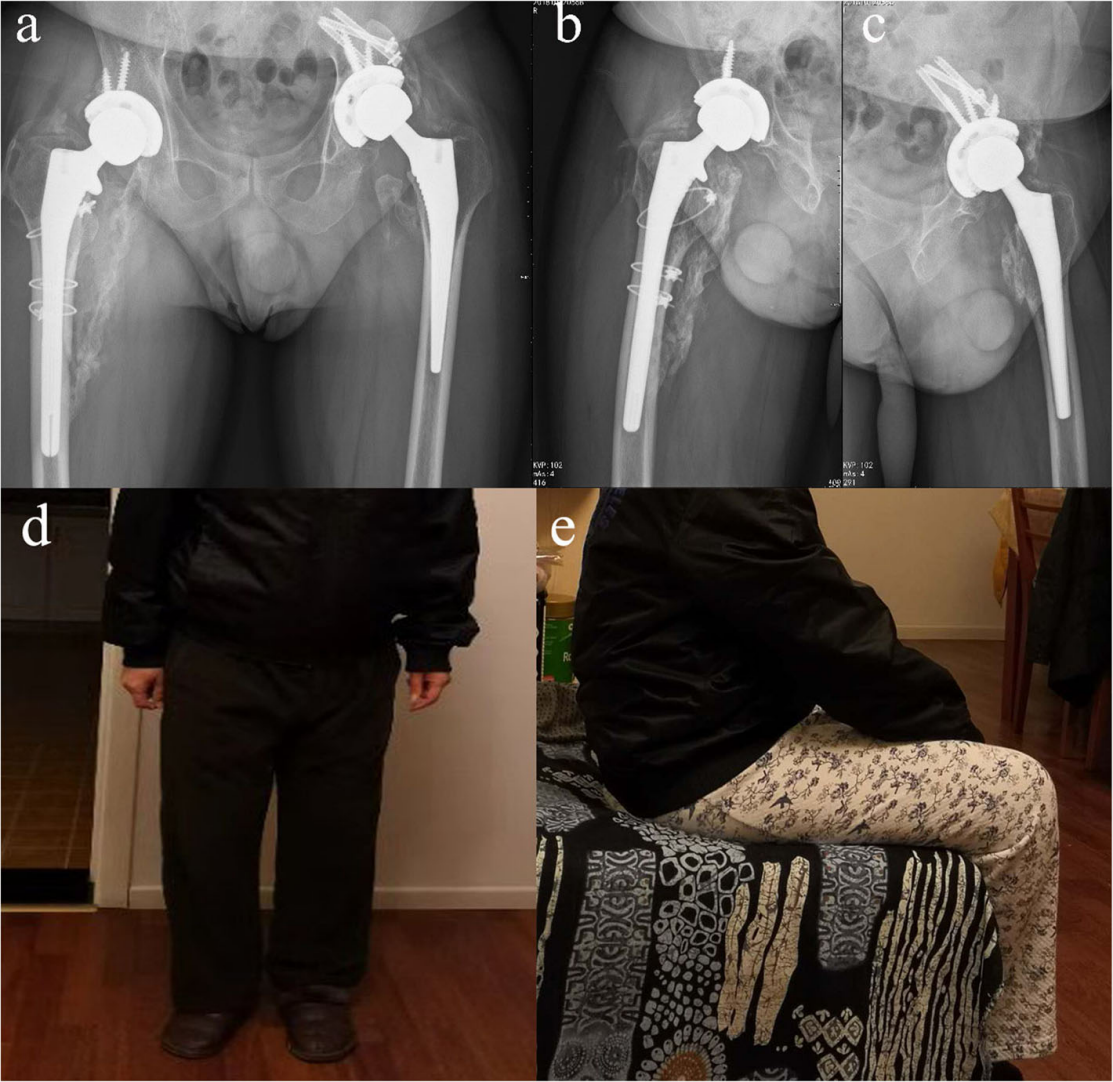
The anterior-posterior (a) and lateral (b & c) radiograph of bilateral hip joints, which taken at 6th month after bilateral THA revision, showed the good position of bilateral prostheses, healing of bone graft on the right side and bilateral ossifying myositis around hip joints. The patient restored a partial range of motion of injured hip joints at the 6th month after bilateral revision
